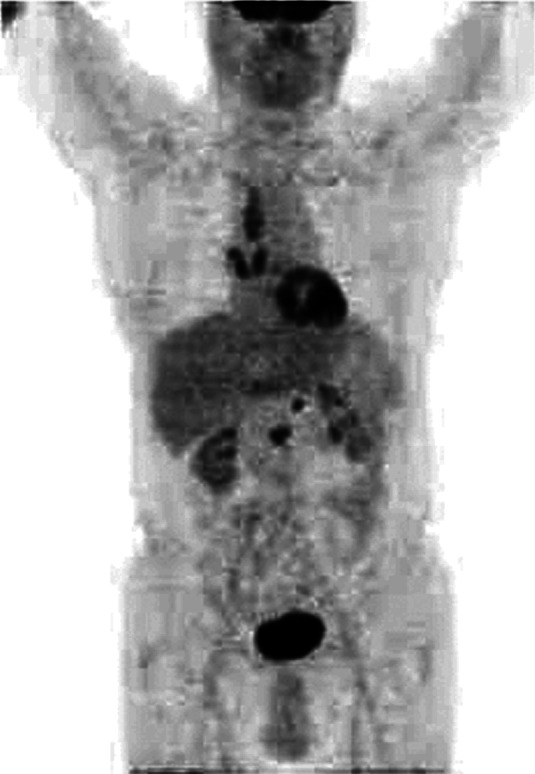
[^18^F]FDG-PET/CT image of a 67-year-old male patient, 2 years after the initial treatment. The [^18^F]FDG-PET/CT performed to assess for suspected recurrence showed increased uptake of [^18^F]FDG in the mediastinal lymph nodes (SUVmax = 6.1), including the right paratracheal, precarinal, subcarinal and right hilar lymph nodes (SUVmax = 7.9), a left superior mesenteric lymph node (SUVmax = 5.5) and the body of the second lumbar vertebra (SUVmax = 6.9). The previously performed CT had not revealed the lesions in the vertebra and the mesenteric lymph node. FDG-PET/CT, fluorodeoxyglucose-positron emission tomography/computed tomography; SUVmax, maximum standardized uptake value.
